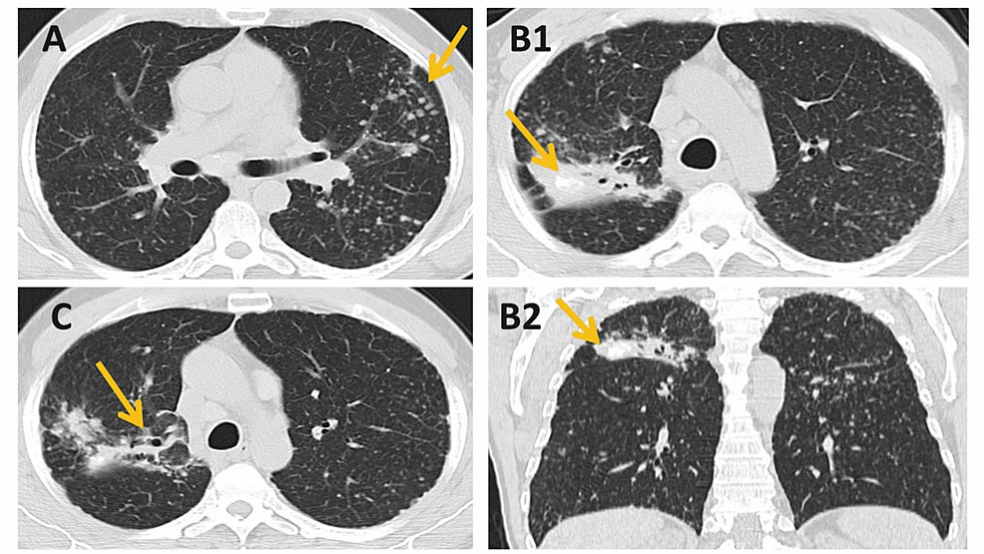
Thoracic computed tomography A - pattern of diffuse centrilobular micronodularity (arrow); B - a consolidative area in the posterior segment of the upper lobe of the right lung, with air bronchogram and calcifications inside (arrow); B1 - axial plane; B2 - coronal plane; C - small bronchiectasis associated with an area of ​​consolidation (arrow)
